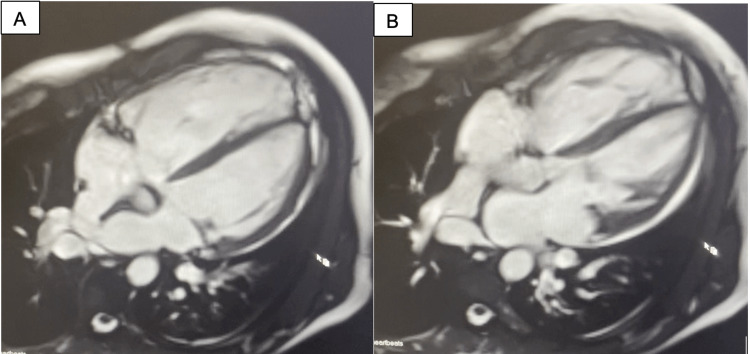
Cardiac MRI showing sinus venosus ASD (A) and partial anomalous venous return of the right upper and middle pulmonary veins into the right atrium (B) MRI: magnetic resonance imaging, ASD: atrial septal defect
